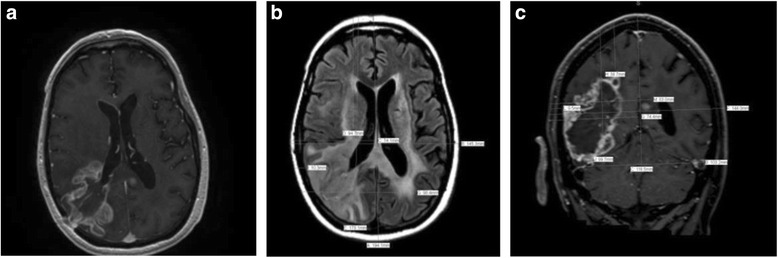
a, b & c demonstrate treatment planning for a recurrent glioblastoma patient with diffuse infiltrating disease. Figure a shows the T1 with contrast axial slice demonstrating enhancing tumor. Figure b demonstrates more diffuse FLAIR signal abnormality, including within the contralateral lobe. As such, treatment is planned using the FLAIR sequence. Standard measurements were performed from a reference frame drawn at the outer margin of the scalp. Tumor location coordinates are obtained on the axial slice measuring from the right frame to the proximal and distal extents of FLAIR abnormality. In the absence of a coronal FLAIR sequence, coronal treatment planning is performed using the T1 postcontrast sequence (figure c). In this slice, the inferior margin of the reference frame is drawn to the lowest visible level of tentorium
